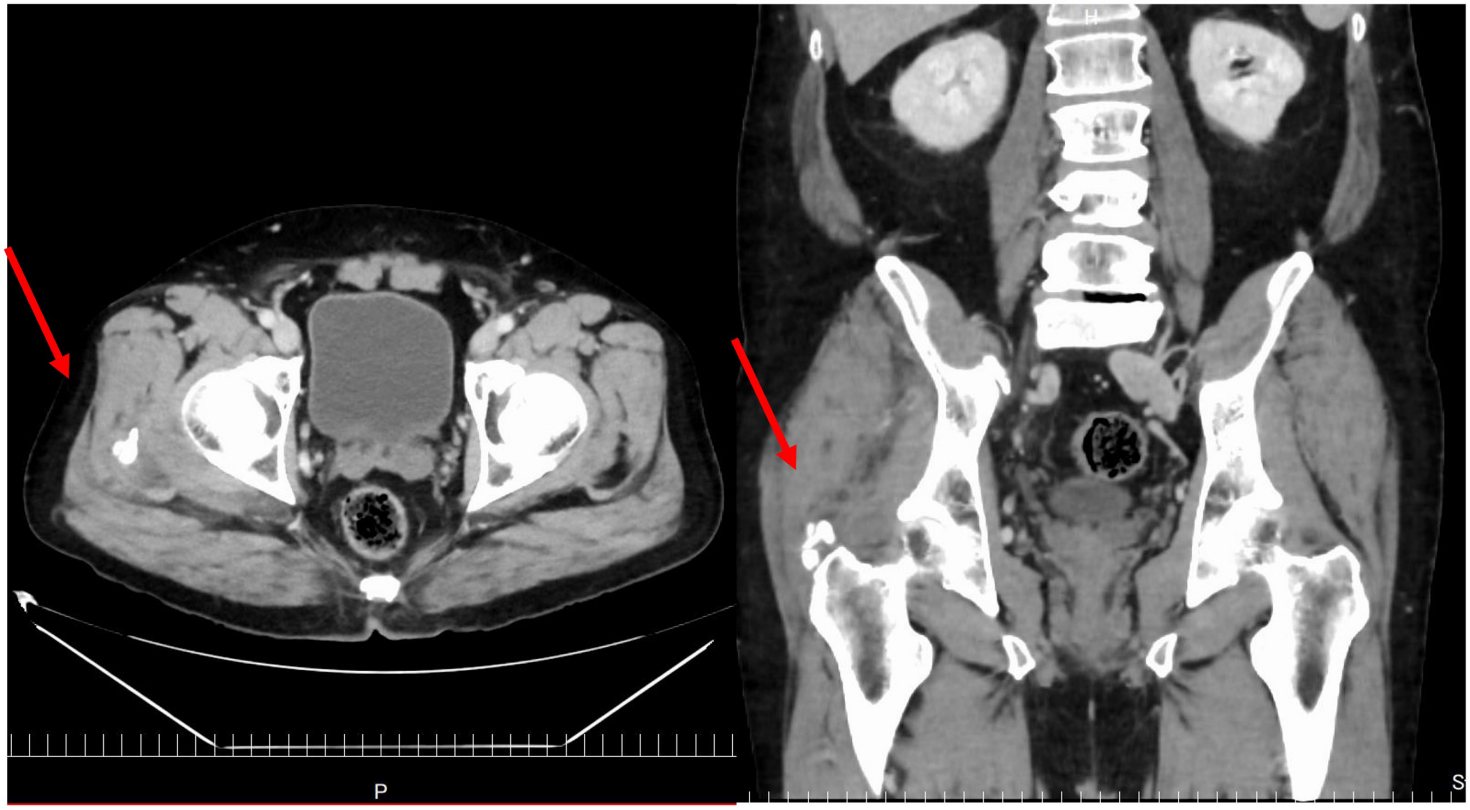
Left; axial: right; coronal; red arrow pointing at the calcification. Computed tomography scan with contrast showed a calcification (1.5 cm x 1.7 cm) slightly proximal to the right femoral tuberosity and edema of the surrounding soft tissues, including the gluteus medius muscle.
